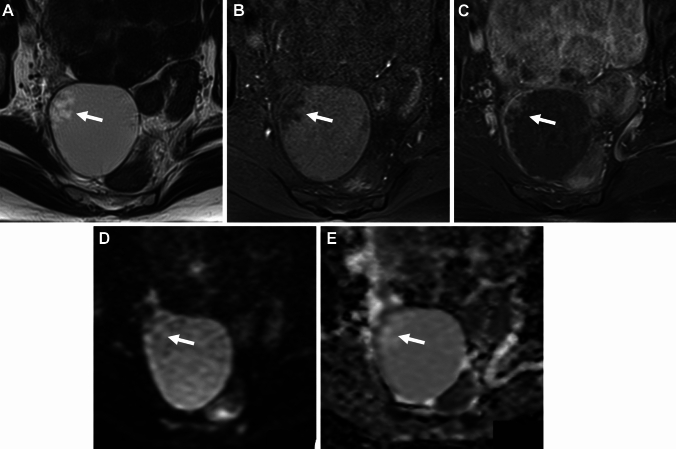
Endometriosis-related ovarian neoplasm: seromucinous borderline tumor. A 55-year-old with suspicion of an ovarian cystic mass with mural nodules on ultrasonography examination. A T2-weighted image, B fat-saturated T1-weighted image, C contrast-enhanced subtraction image, D DWI (*b* = 800 s/mm^2^), E ADC map show an endometrioma with a papillary mural nodule (arrow). The papillary mural nodule shows high signal intensity with low signal intensity dendritic fibrous core on T2-weighted image, relatively weak contrast-enhancement, and high signal intensity on DWI with high ADC (T2 shine-through)
